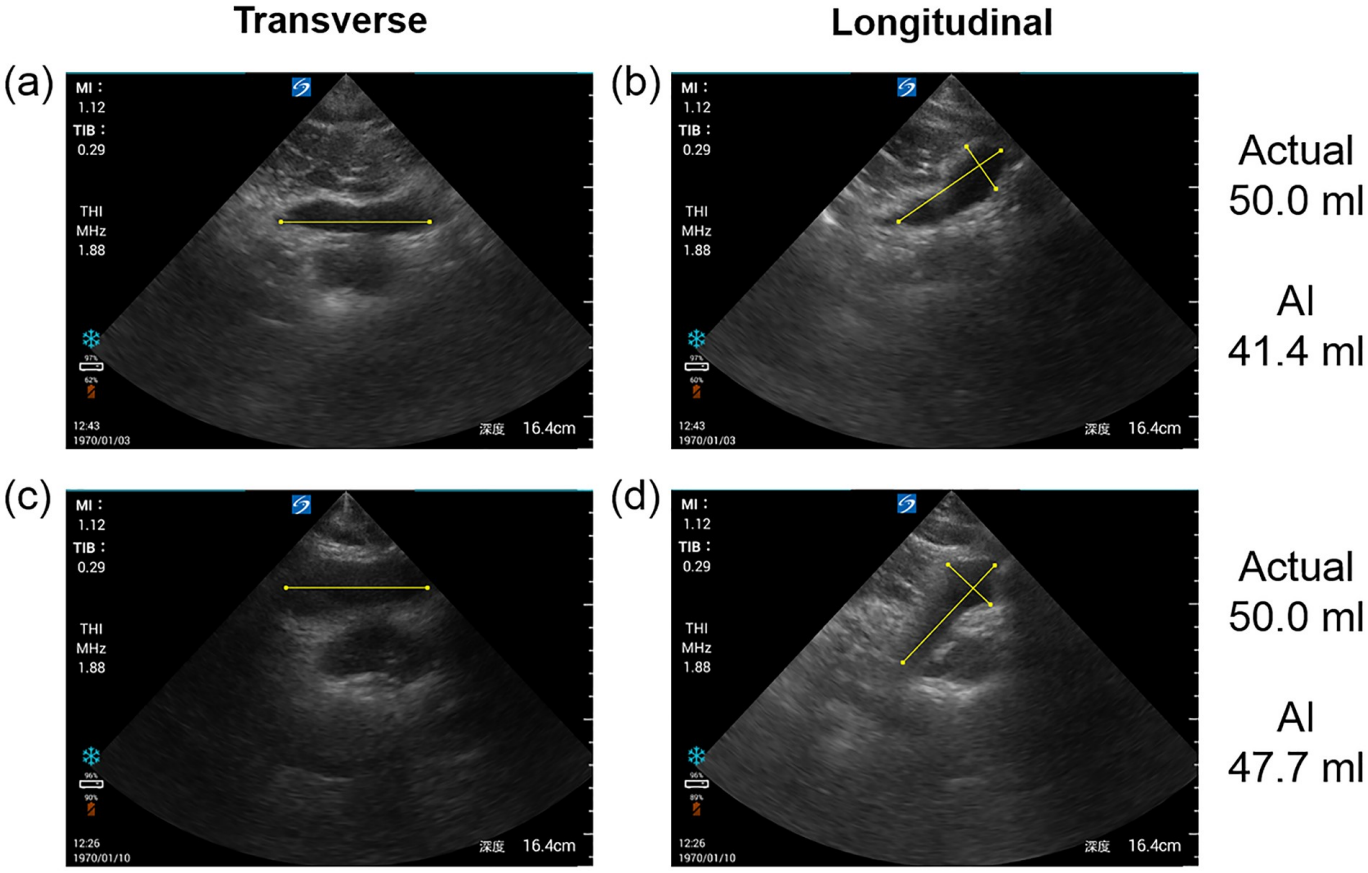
Two cases of false negatives. (a, b) Transverse and longitudinal ultrasound (US) images of the same male aged approximately 20 years. (c, d) transverse and longitudinal US images of the same male aged approximately 30 years. Actual: Urine volume actually urinated by the subject. AI: Value of bladder urine volume estimated by automatic estimation tool. Yellow lines are detected by AI as bladder diameters.
